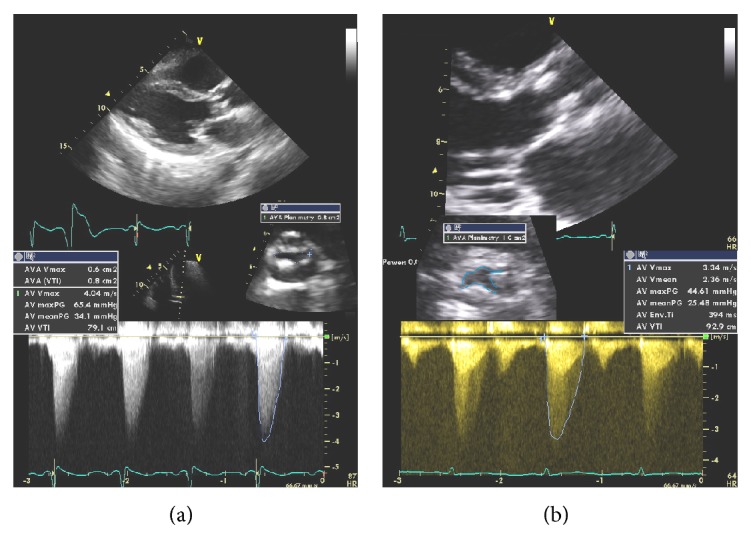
Transthoracic echocardiography before and after valvuloplasty shows a calcified, stenotic aortic valve with AVA of 0.8 cm^2^ by planimetry and continuity equation (VTI). Postvalvuloplasty there is demonstrated improvement in aortic valve gradients as well as AVA.
